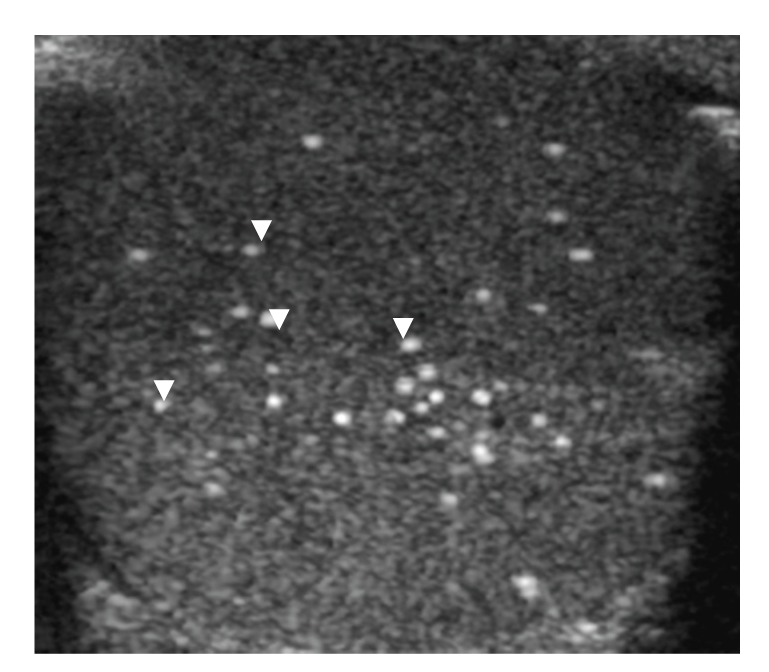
Grey scale transcrotal axial ultrasound of another patient with testicular microlithiasis. Note the multiple tiny densely echogenic, punctate and well-defined lesions (white arrowheads) easily distinguishable from those in testicular hamartomas in Figure 1.
